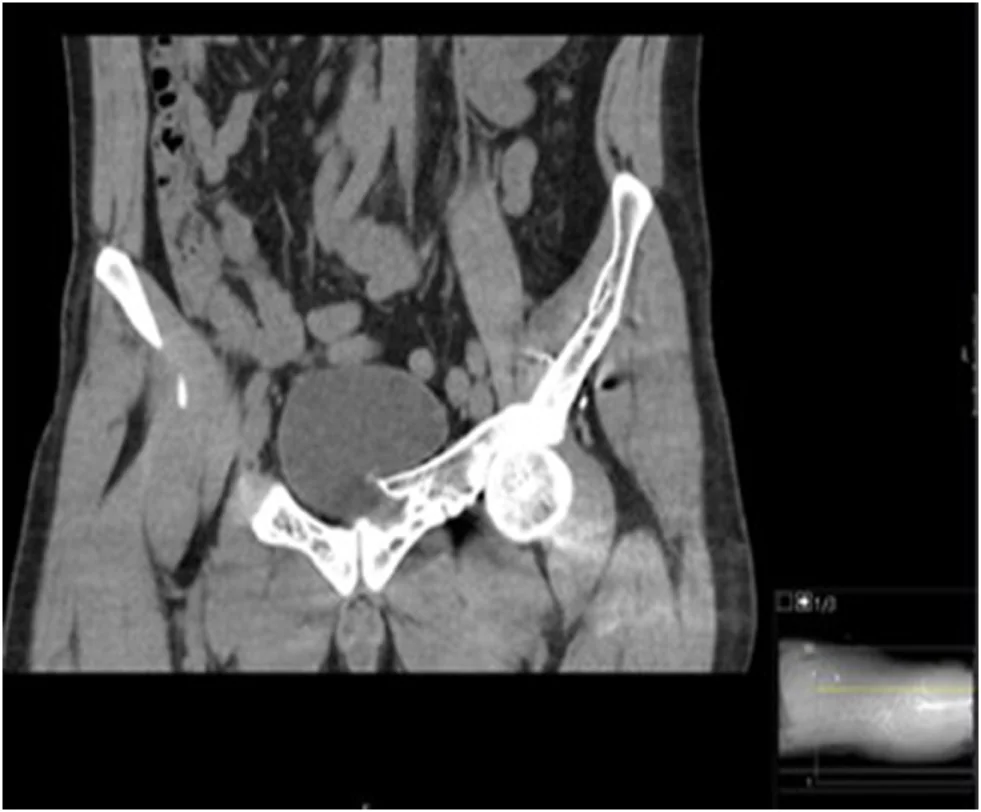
Pelvic CT (coronal reformation) demonstrates mature sclerotic heterotopic ossification arising from the left ischium and extending toward the urinary bladder, with retained cephalomedullary (gamma) nail and iliac stabilization screws from prior pelvic reconstruction.
